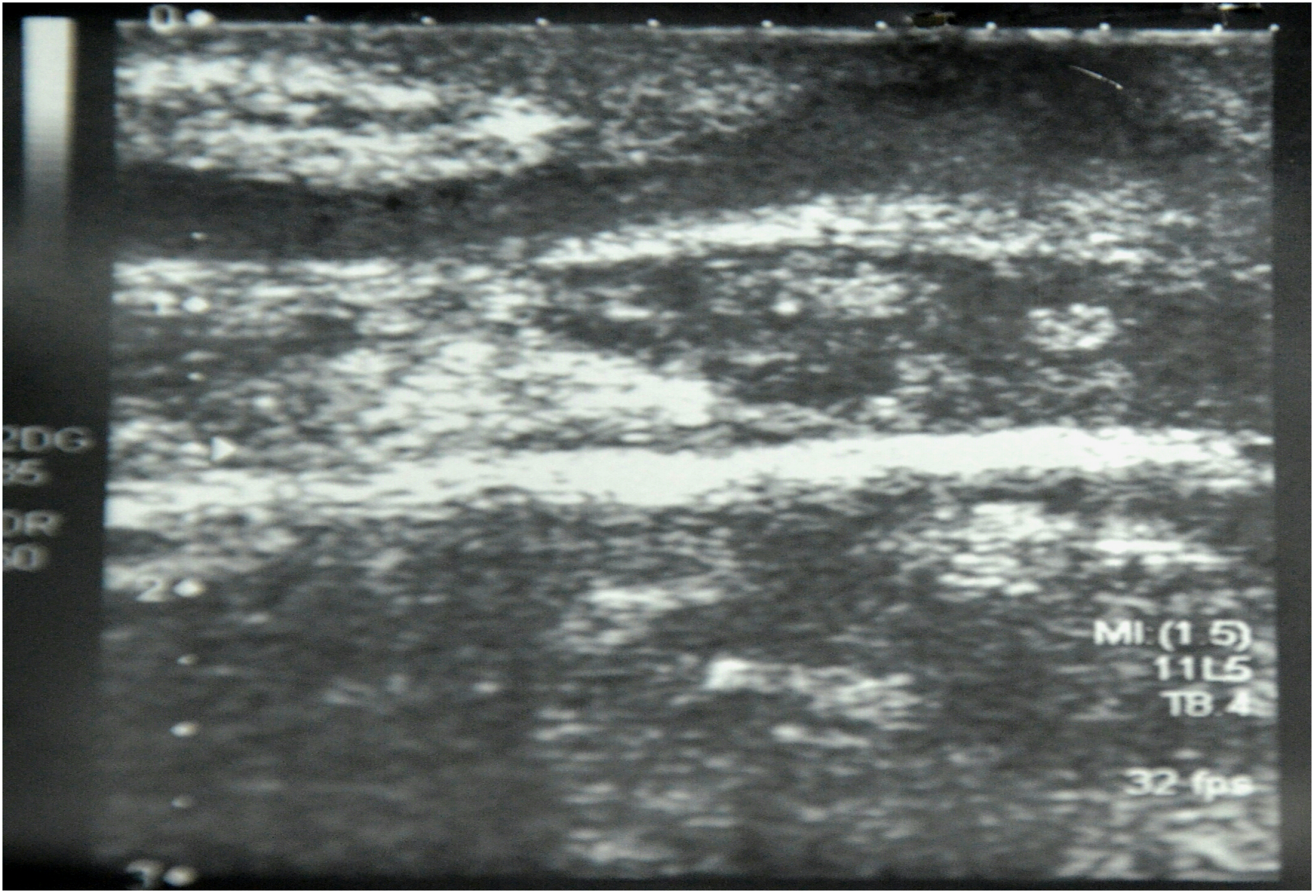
Ultrasound scan showing multiple thick-walled pockets containing multiple echogenic grains, with minimal subcutaneous oedema.
